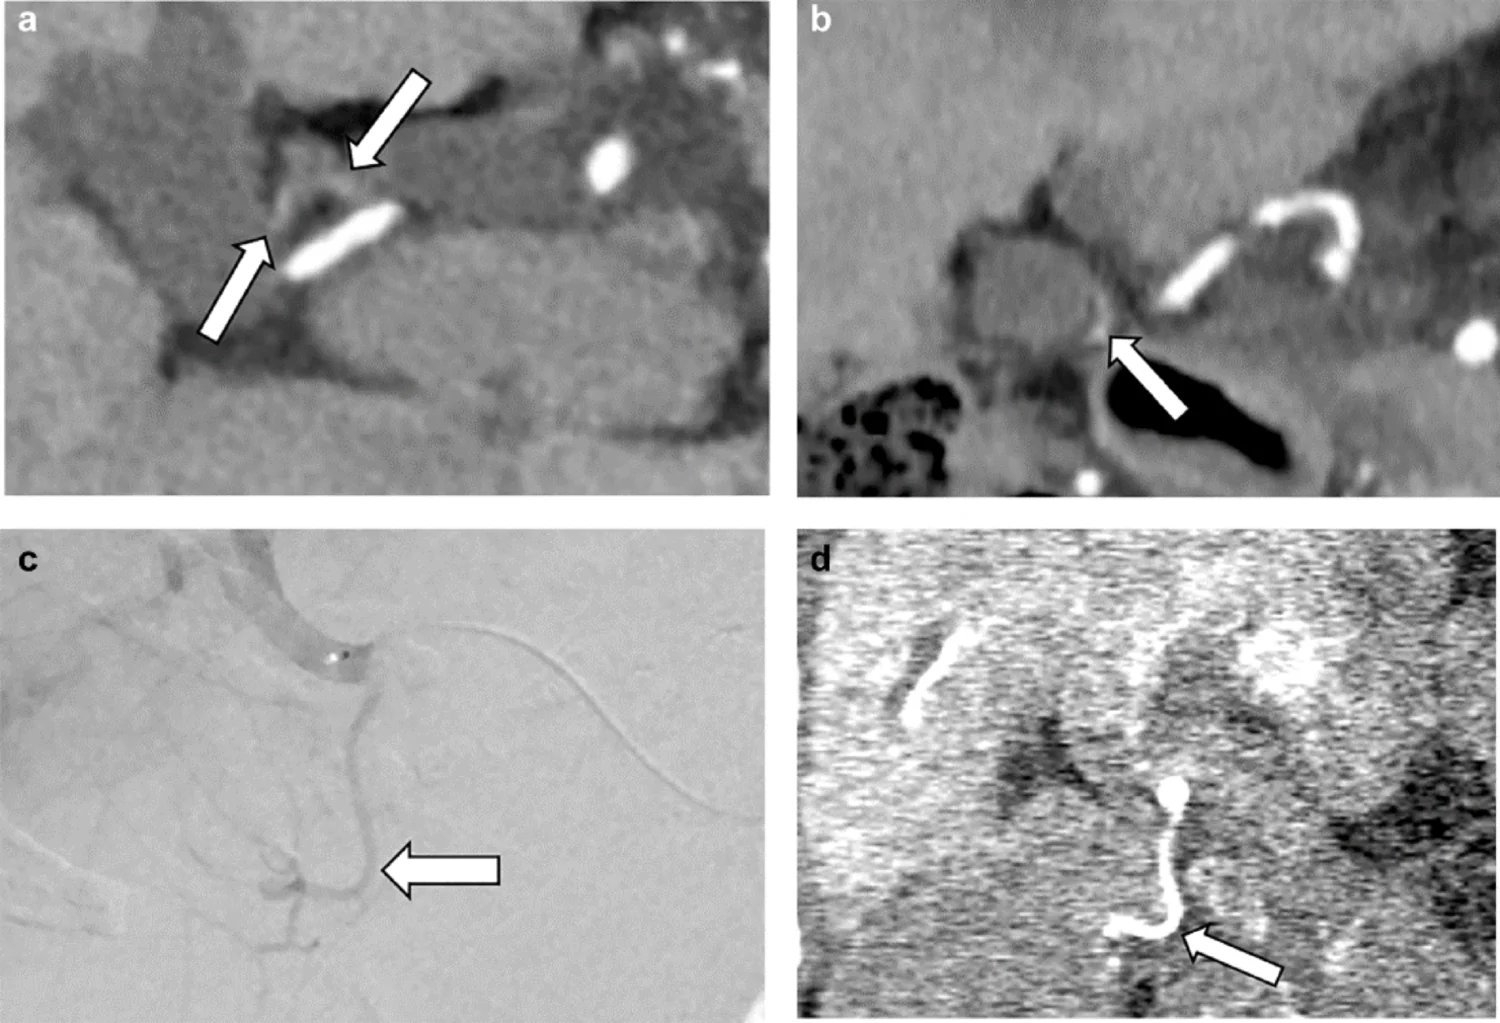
An 83-year-old woman undergoing treatment for hepatocellular carcinoma underwent abdominal angiography 6 days after contrast-enhanced CT. The arrows indicate the cystic artery. A single cystic artery was identified on the arterial-phase contrast-enhanced CT cross-sectional images (a) and coronal images (b) reconstructed with SR-DLR. A single cystic artery was also confirmed on right hepatic artery angiography (c) and intraoperative CT during hepatic arteriography (d)
